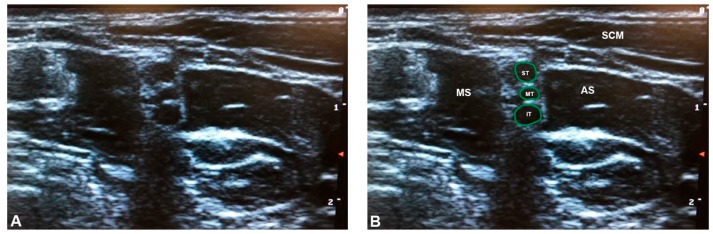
The classic brachial plexus anatomy identified on ultrasound: (A) unlabeled; and (B) labeled. Note that the superior, middle, and inferior trunks are clearly separated from the anterior and middle scalene muscles by hyperechoic fascial planes. AS = anterior scalene; IT = inferior trunk; MS = middle scalene; MT = middle trunk; SCM = sternocleidomastoid; ST = superior trunk. The green outlines demarcate the trunks of the brachial plexus.
